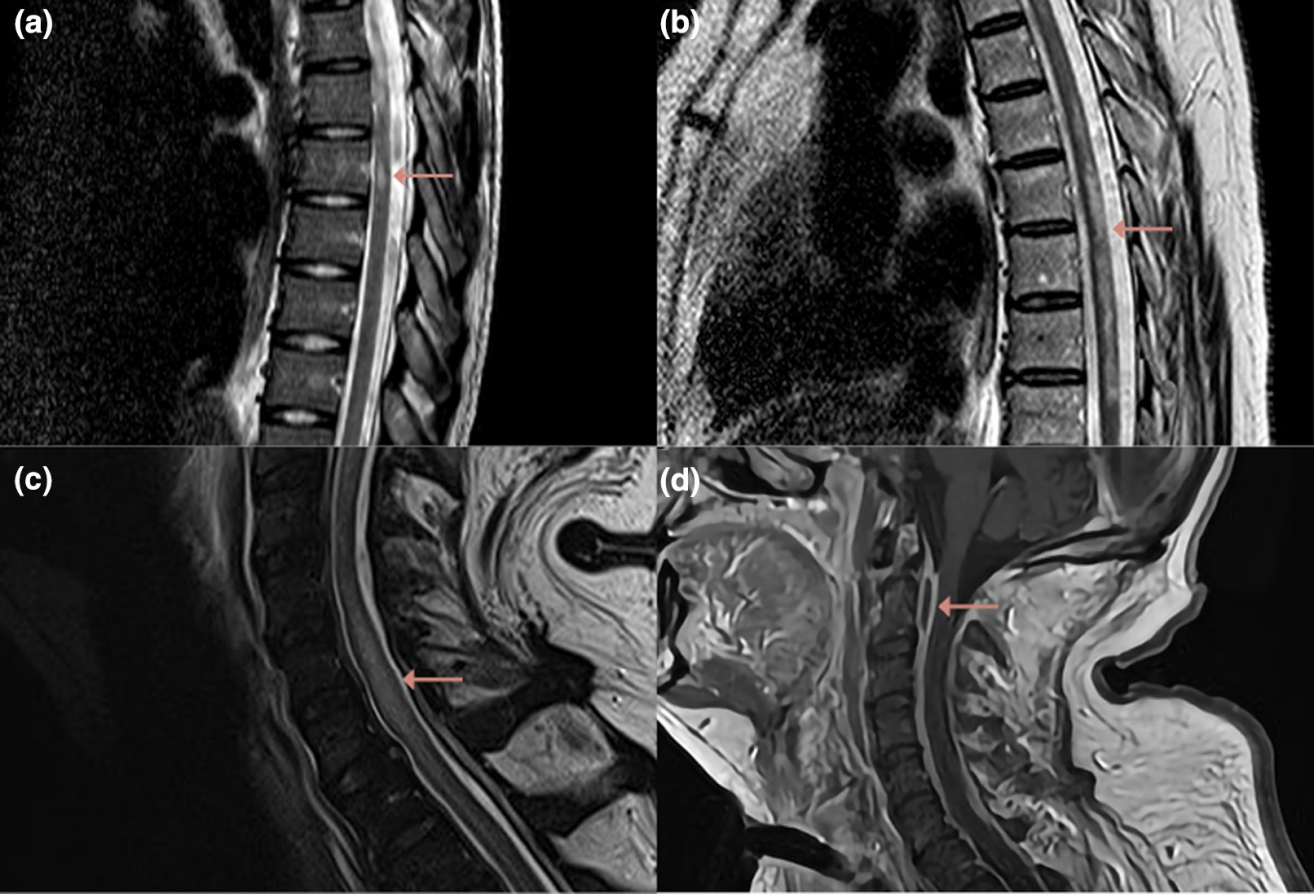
Spinal magnetic resonance imaging (MRI) images of patients with noncompressive myelopathy secondary to bacterial meningitis. (a) T2‐weighted sagittal spinal MRI shows an hyperintense lesion at T6–T7. This patient was diagnosed with postinflammatory myelitis. (b) T2‐weighted sagittal spinal MRI shows a longitudinal hyperintense lesion in a large part of the thoracic spinal cord. This patient was also diagnosed with postinflammatory myelitis. (c, d) T2‐weighted sagittal spinal MRI shows a longitudinal hyperintense lesion in the lower cervical spinal cord (c) and an epidural abscess at C1–C2 (d) on T1‐weighted images. This patient was diagnosed with infectious myelitis.
